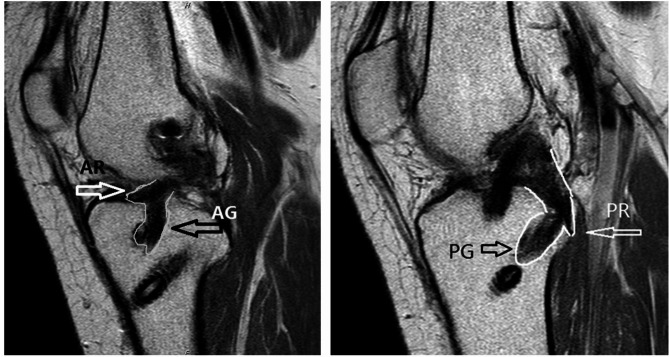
MRI images revealed good fusion of ligamentous remnant with anterior cruciate ligament graft (AG) and posterior cruciate ligament graft (PG) after the surgery
